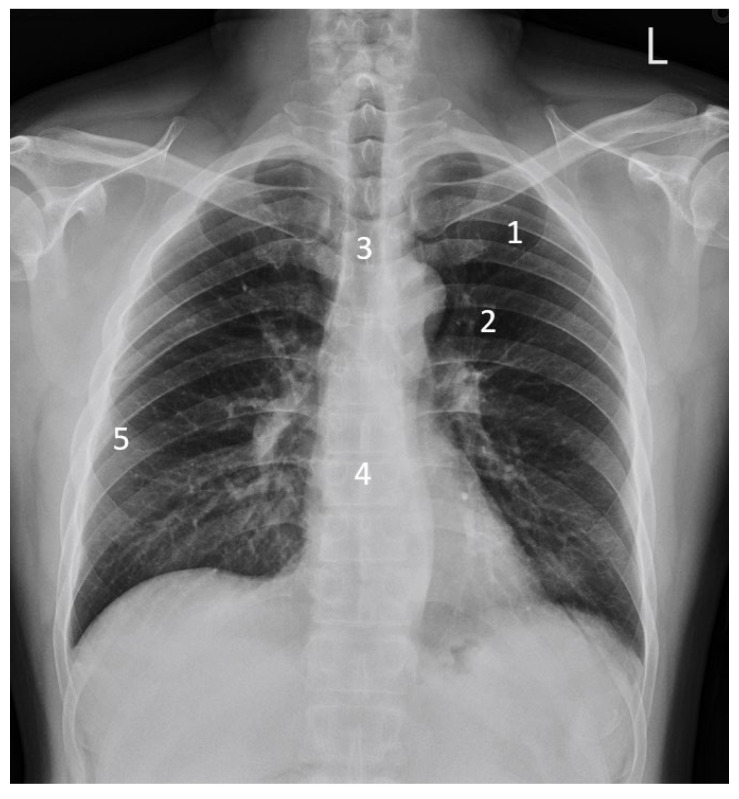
The five body structures assessed using visual grading analysis of chest radiographs. The image was acquired from a 42-year-old male subject with body thickness of 22.03 cm, BMI of 27.78 kg/m^2^, and EI of 160. The subject had a mean VGA score of 3.6 and 4.0 by the two raters. (1) The vessels 3 cm away from the pleural margin. (2) Vessels in the central region. (3) The carina with the main bronchi. (4) The thoracic vertebrae behind the heart. (5) The pleural margin.
